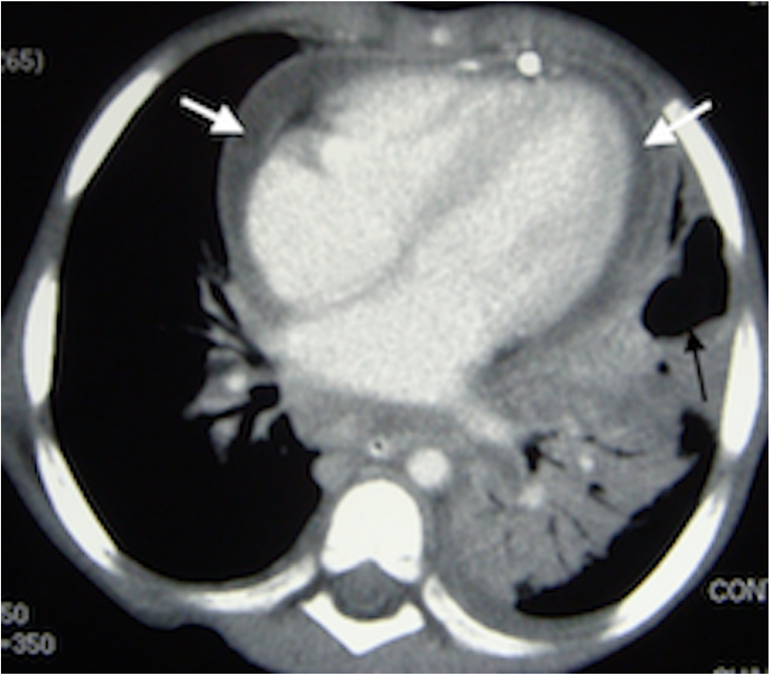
Axial contrast-enhanced CT in a 2-year-old girl with left-side necrotising pneumonia and pericardial collection (*white arrows*) in the process of being drained. There is poor enhancement of the visible portions of the left lung and an air-filled thin-walled cavity without surrounding enhancement representing cavitary necrosis (*black arrow*)
